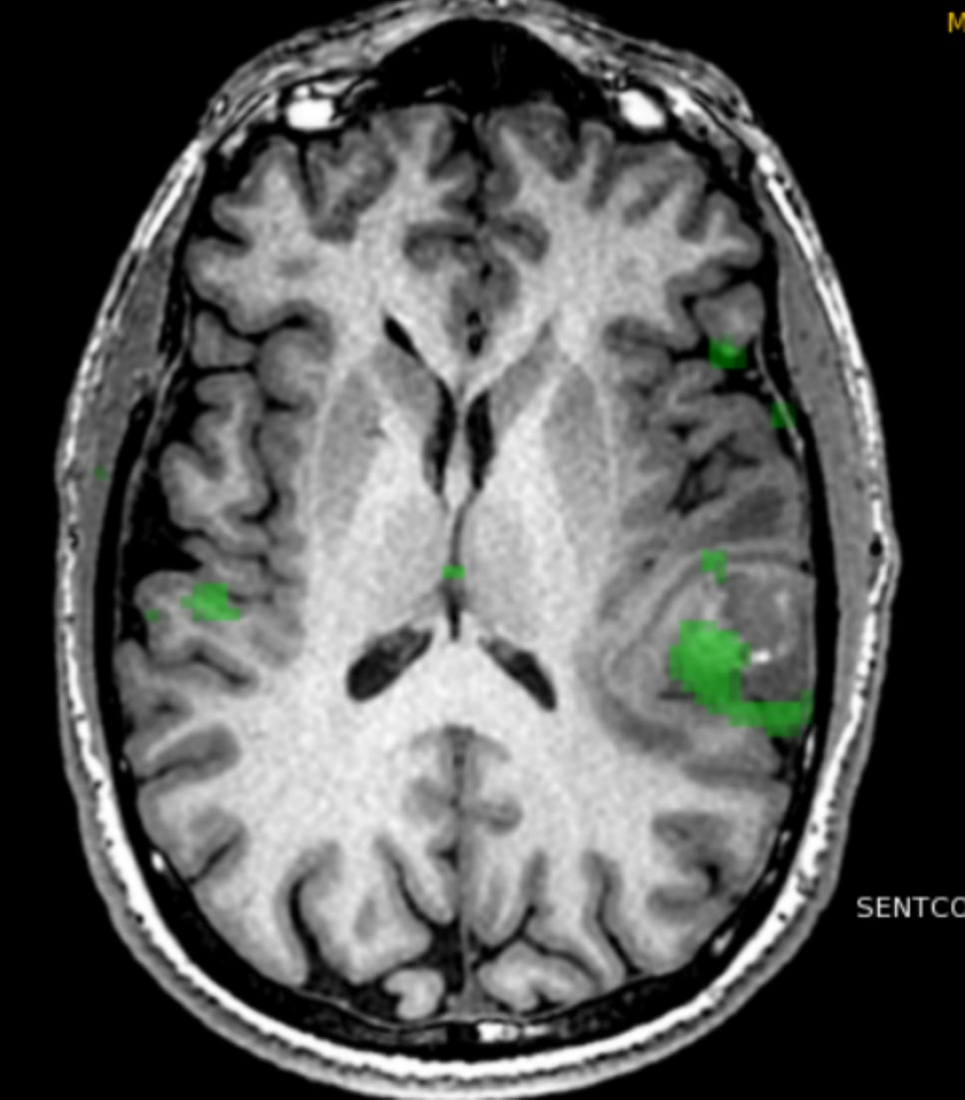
Patient with a glioblastoma in the left temporal lobe. Functional MRI depicting left sided language lateralization
